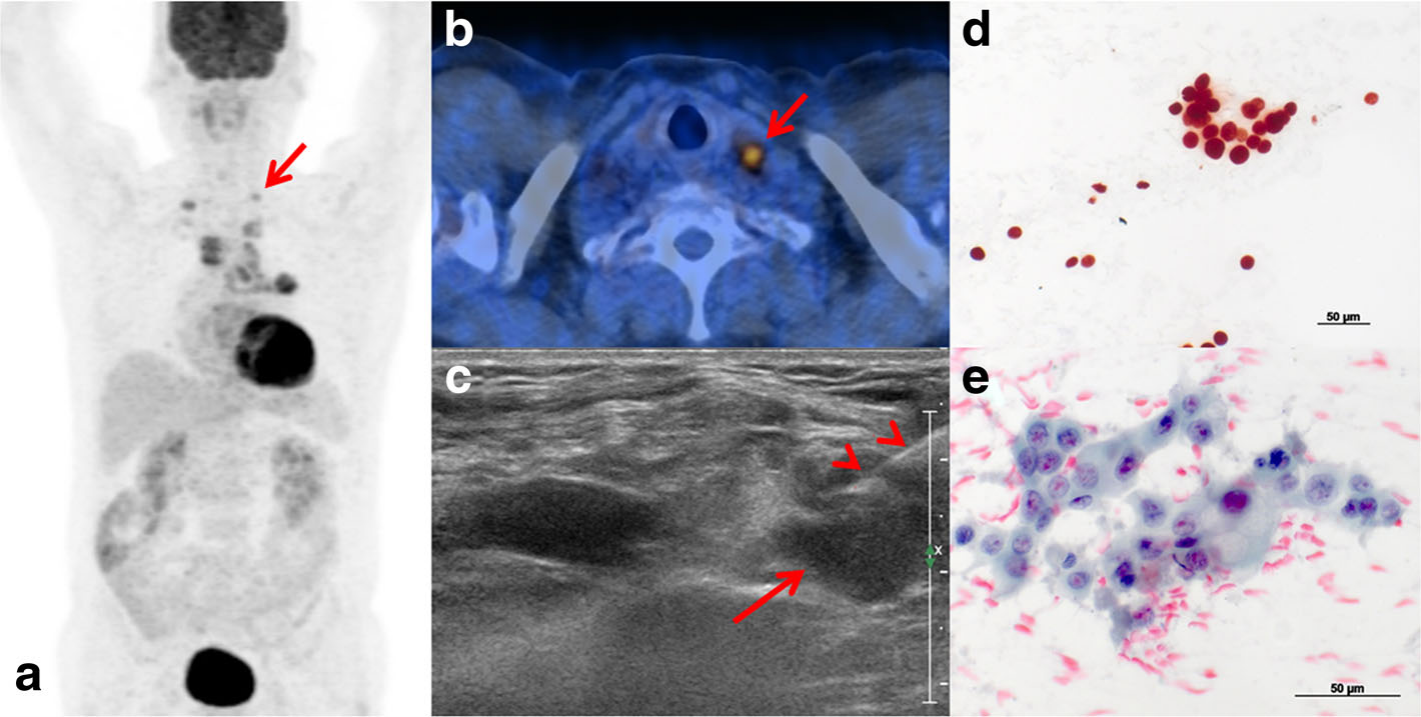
FDG PET/CT of a patient with suspected lung cancer. Bronchoscopy performed in an external hospital was not diagnostic. MIP image a shows a primary tumor in the left lung with bilateral FDG active mediastinal and supraclavicular lymph nodes (*arrow*). Axial fused PET/CT b and ultrasound c showed a SCLN (*arrow*) which was biopsied with fine and core needle (*arrowheads*). Cytology showed TTF 1 positive d adenocarcinoma (e). In this case no driver mutation identified
